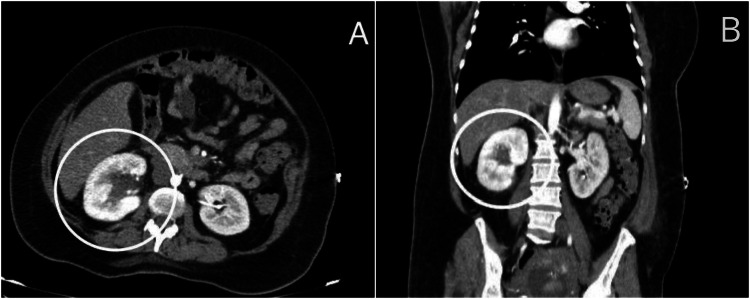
Renal Angio-CT, arterial phase, axial (A) and coronal (B) slices, showng a delay in the nephrogram of the left kidney compared to the right kidney, compatible with contralateral compensatory phenomenon due to the inflammatory process in the right kidney. Right kidney pyelocaliceal dilatation, evident in both slices, which may be related to an obstructive process or complicated infection, perirenal fat streaking characteristic of changes consistent with an inflammatory process.
